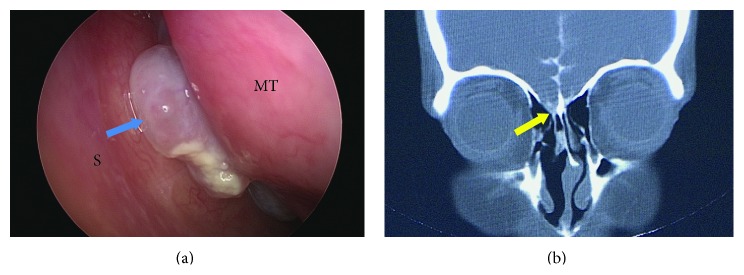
Patient number 2. (a) Left meningocele (arrow) between the nasal septum (S) and middle turbinate (MT). (b) CT myelography showing a defect at the level of the cribriform plate (arrow head).
